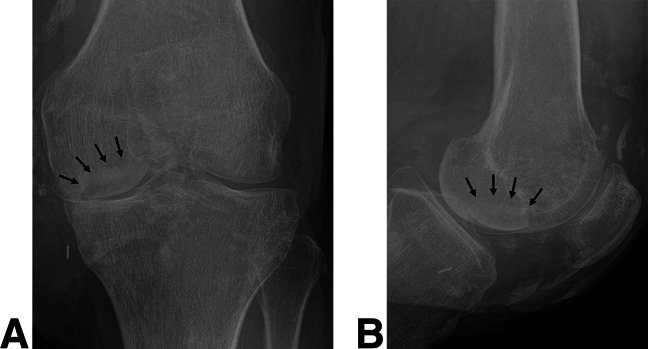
A and B, Orthogonal radiographs 12 weeks postoperatively demonstrating appropriate placement of the bone plug in the lateral aspect of the medial femoral condyle with congruence of the subchondral bone and osseous incorporation at the osteochondral allograft recipient site interface (arrows).
